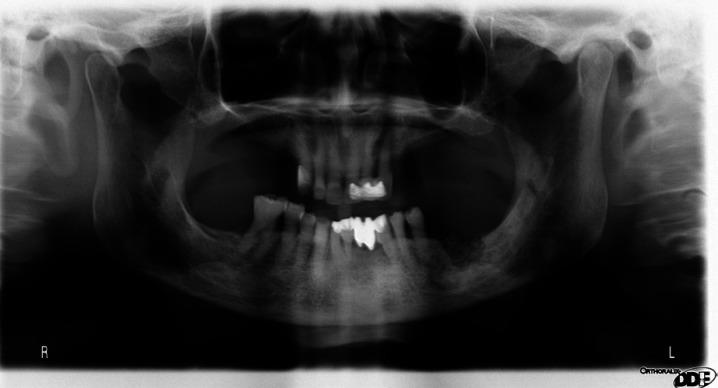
Orthopantomography of osteonecrosis in case 5.
